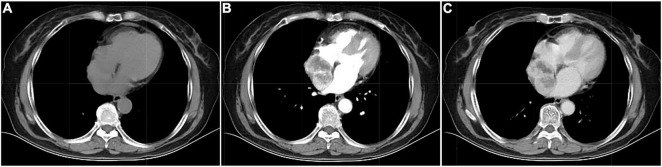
Computed tomography images of cardiac paraganglioma. (A) Plain image; (B) arterial phase image; (C) venous phase image.
